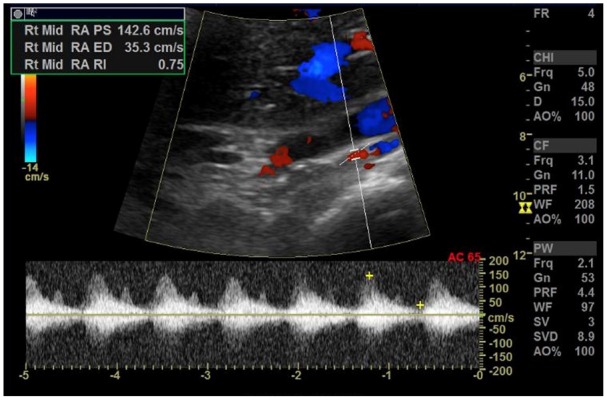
Duplex ultrasound of the right kidney showing patent blood flow to the kidney via the right renal artery.
